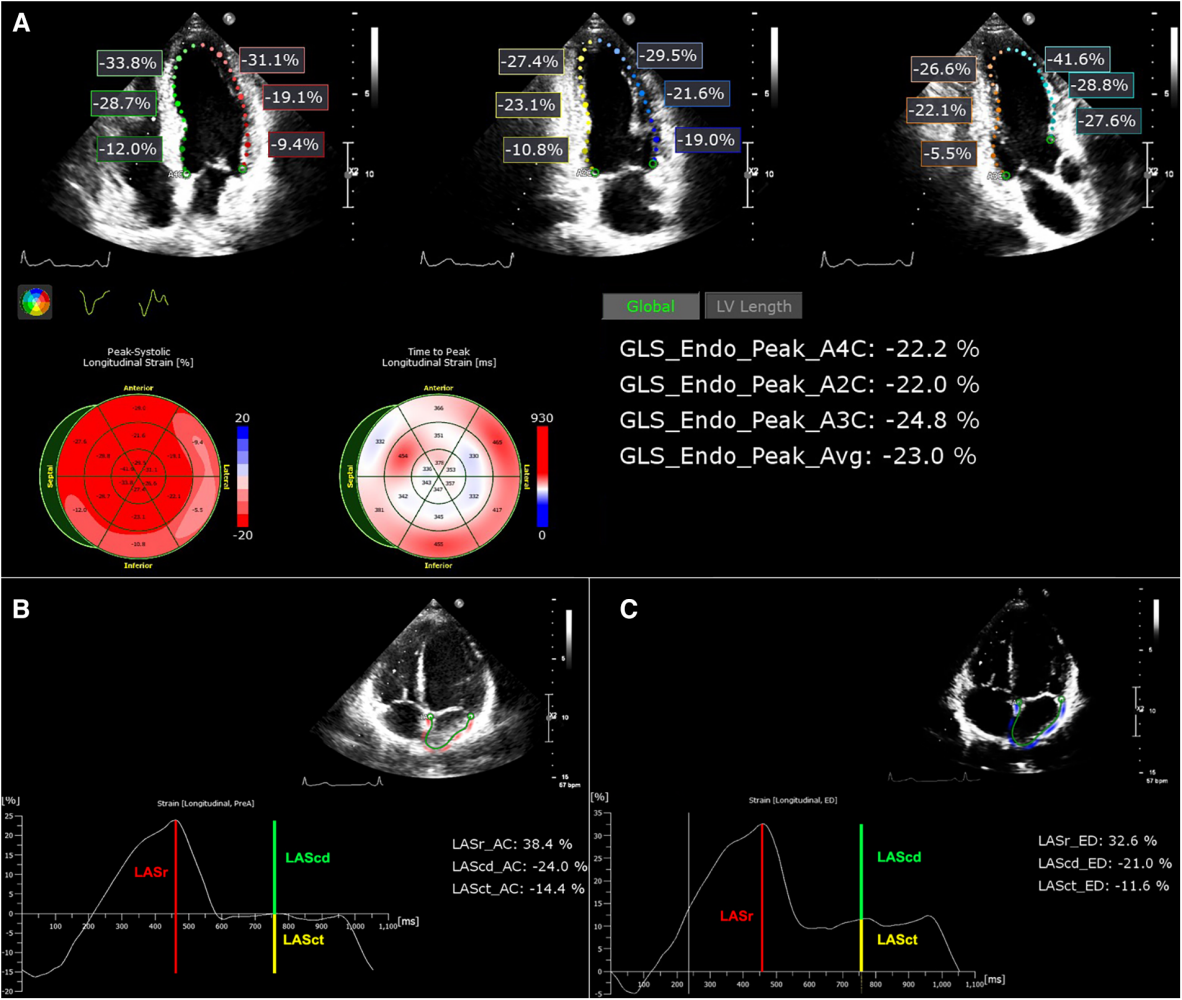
(A) Images of LV strain analysis. The results are displayed with the LV GLS for the apical 4-chamber, 2-chamber, and 3-chamber chambers and their respective averages. (B,C) LA strain images with reference points beginning at the *P* wave and *R* wave, respectively.
